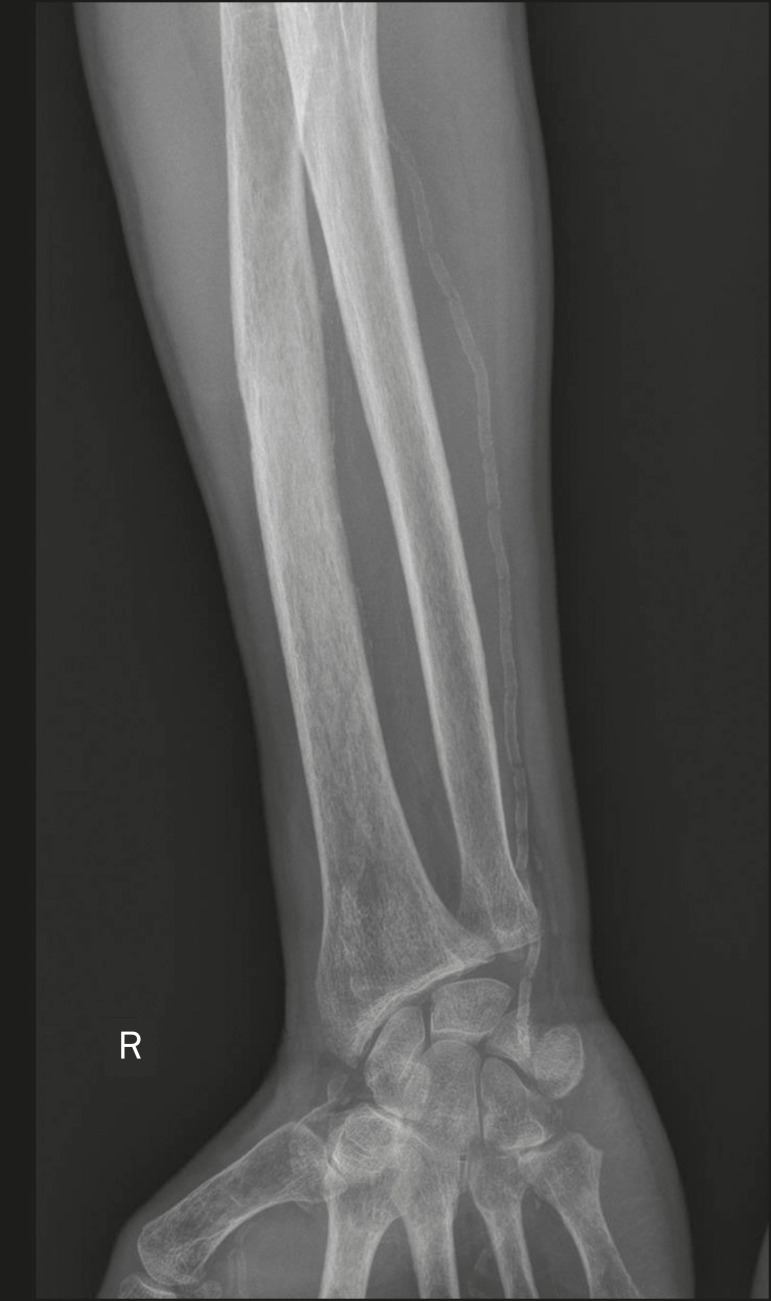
Vascular calcifications in medial calcific sclerosis (Mönckeberg’s arteriosclerosis). Frontal radiograph of the forearm showing small, diffuse, circumferential calcifications in the ulnar artery wall and no luminal narrowing, which is characteristic of Mönckeberg’s arteriosclerosis, caused by calcium deposition in the middle layers of small- and medium-caliber vessels.
